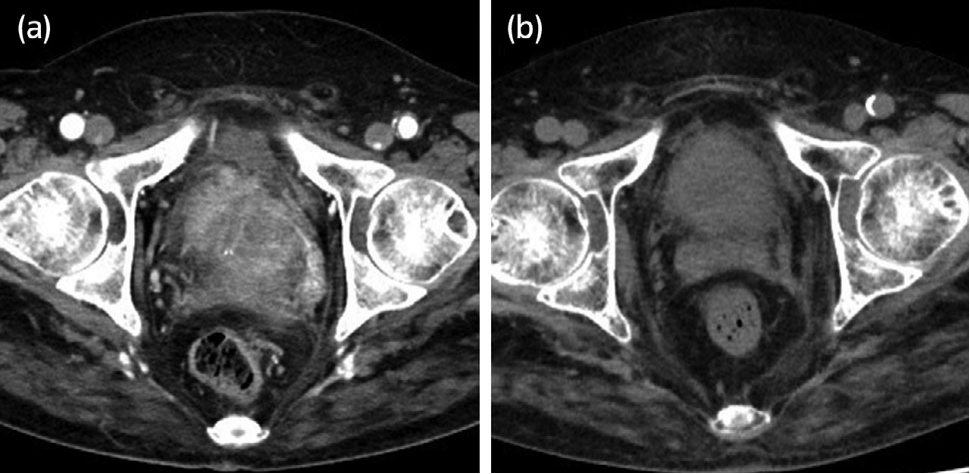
Pelvic CT scans at diagnosis (a) and after initiation of treatment for prostate cancer (b). (a) The contrast‐enhanced CT scan at the diagnosis revealed that the prostate was irregularly enlarged with a diameter of 84 mm, suggesting left seminal vesicle invasion of prostate cancer accompanied by a swelling right obturatorius lymph node. (b) The simple CT scan showed a significant decrease in size of prostate cancer after initiation of dalolutamide intake and radiation therapy to the prostate.
